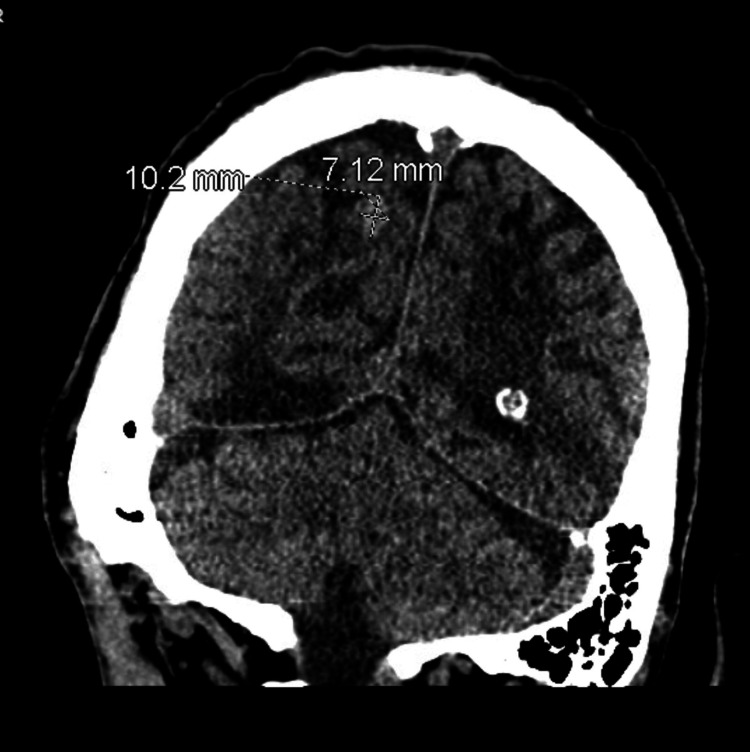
Computed tomography of the brain on day 1 of hospitalization: right parietal intraparenchymal hemorrhage measuring approximately 7 x 10 mm.
